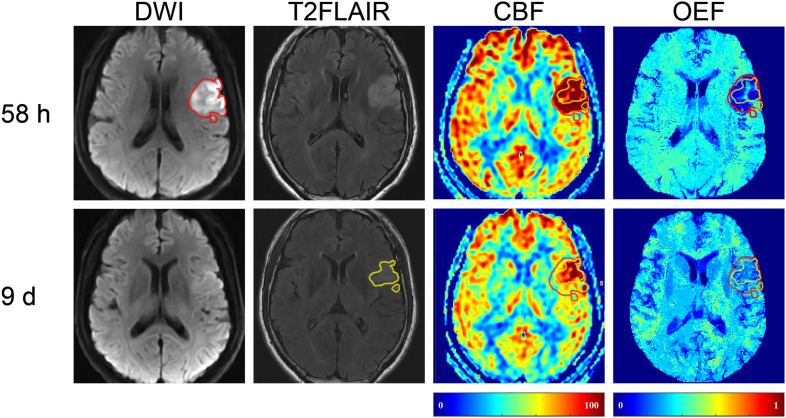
Representative images of a 57-year-old male with right limb weakness. The final infarct (FI, with a volume of 8.3 ml on 9-day T2 fluid-attenuated inversion recovery (T2FLAIR) (shown in yellow) was smaller than the 58-h initial diffusion lesion (IDL, 13.18 ml) on diffusion-weighted imaging (DWI) (shown in red). The area of IDL that did not overlap with FI was the reversal region (RR). DWI, diffusion-weighted imaging; T2FLAIR, T2 fluid-attenuated inversion recovery; CBF, cerebral blood flow; OEF, oxygen extraction fraction.
